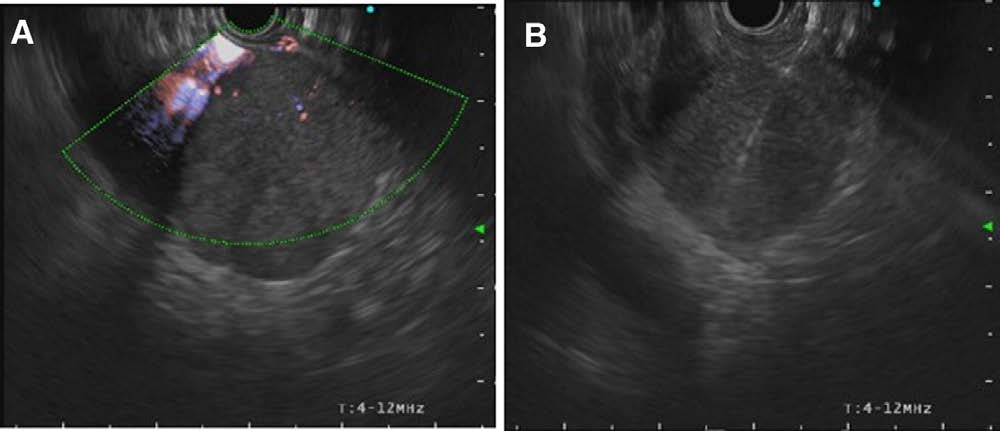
EUS-FNB photo. A relatively well-defined mass of approximately 5 cm in size in the aortocaval space was biopsied using a 22-gauge needle. (2A) Vascularity was confirmed on doppler setting and (2B) needling with a 22-gauge needle. EUS-FNB = endoscopic ultrasound-guided fine-needle biopsy
